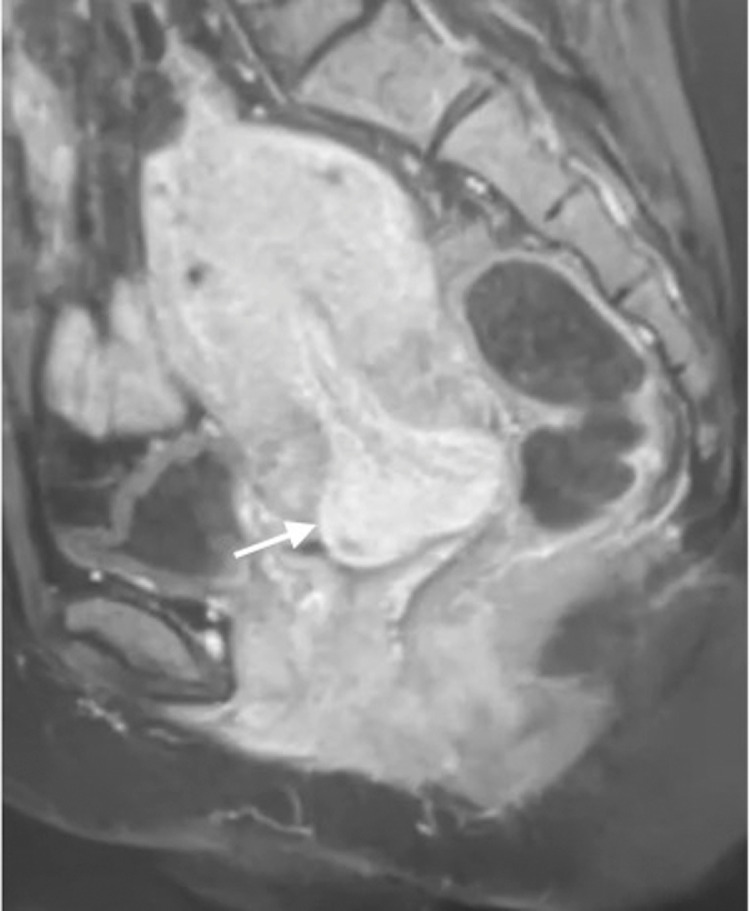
Sagittal plane T1-weighted fat suppressed postcontrast pelvic MR section, showing a voluminous cervical polyp issuing from the cervix, with intense, homogenous contrast enhancement (white arrow).
